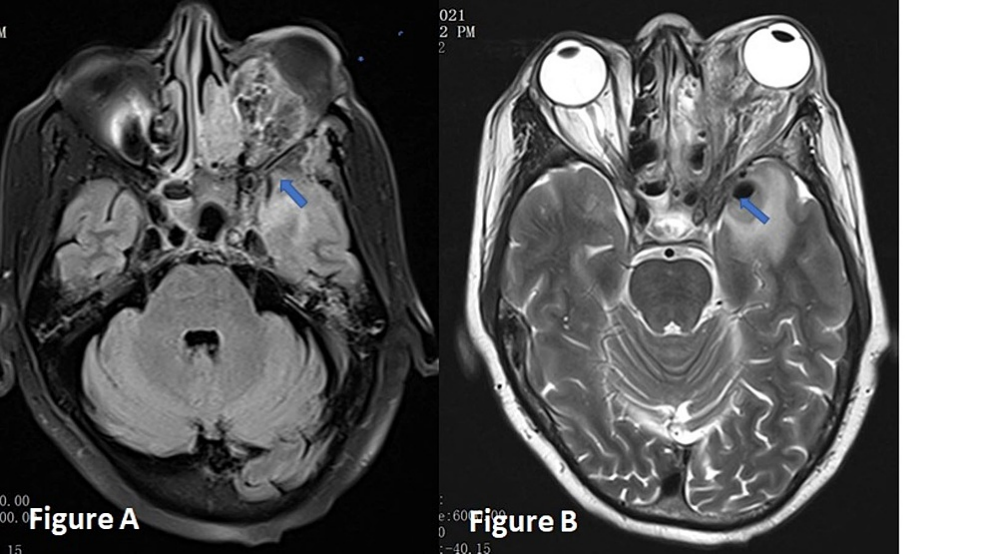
A and B, Radiological images (cranial MRI) of invasive rhino-oculo-cerebral mucormycosis. A: Axial FLAIR, soft tissue swelling in the left orbital region (arrow indicates extension to the left cavernous sinus). B: Axial T2, further deeper extension of the lesion (arrow indicates left pterygopalatine fossa involvement). MRI, magnetic resonance imaging; FLAIR, fluid-attenuated inversion recovery.
